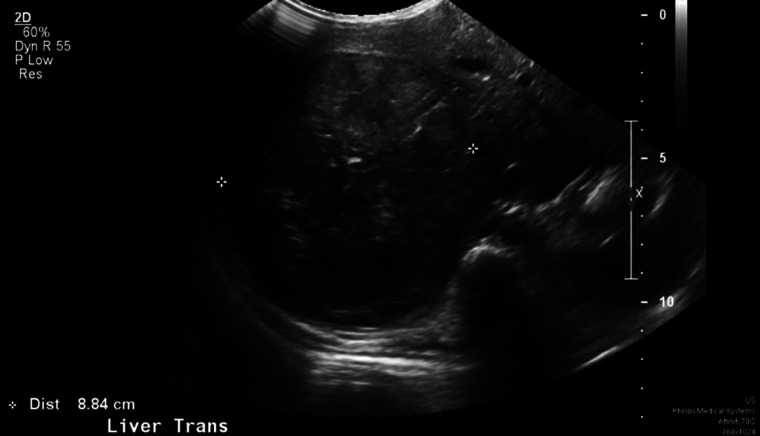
An ultrasound of the patient's abdomen showing a well-circumscribed, 11 × 10 × 10 cm solid mass occupying the right hepatic lobe.
